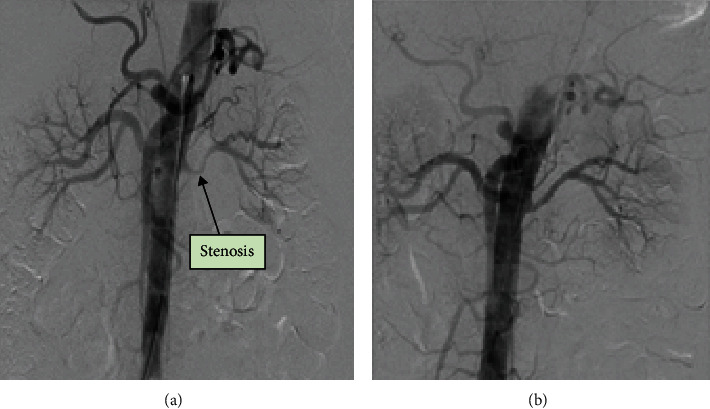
Case 1—(a) CT renal angiogram showing right renal artery before angioplasty. (b) CT renal angiogram showing right renal artery after angioplasty.
